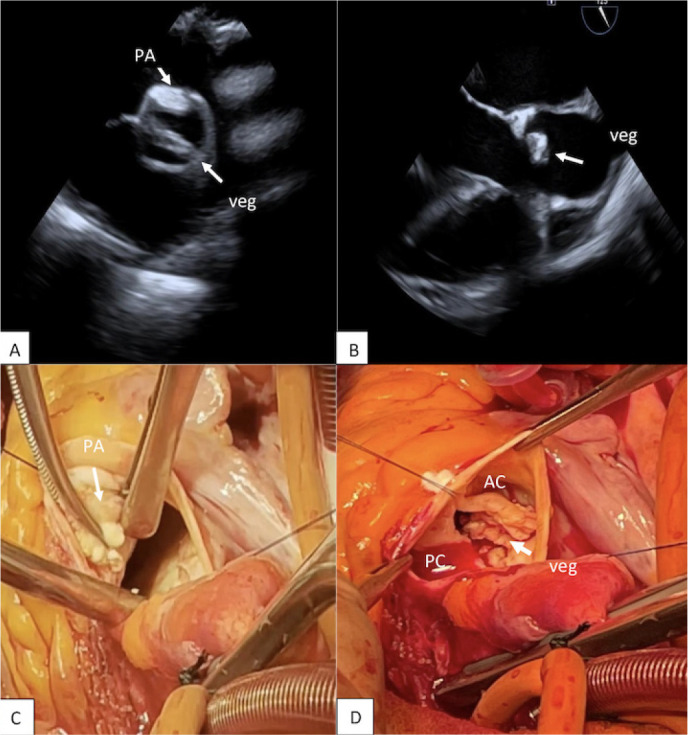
A) Preoperative transesophageal echocardiography (TTE), short
